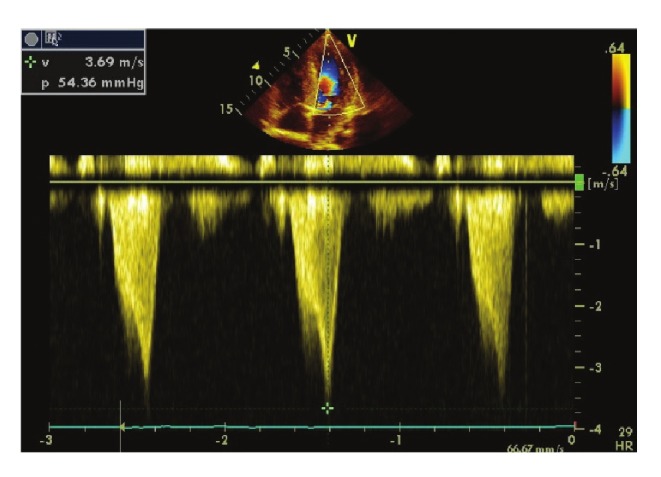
Image d’échographie–Doppler cardiaque coupe apicale 4 cavités d’un patient de 27 ans porteur d’une cardiomyopathie hypertrophique obstructive. On note la présence d’un gradient intra–ventriculaire gauche mesuré à 54.3 mmHg en forme de lame de sabre.
